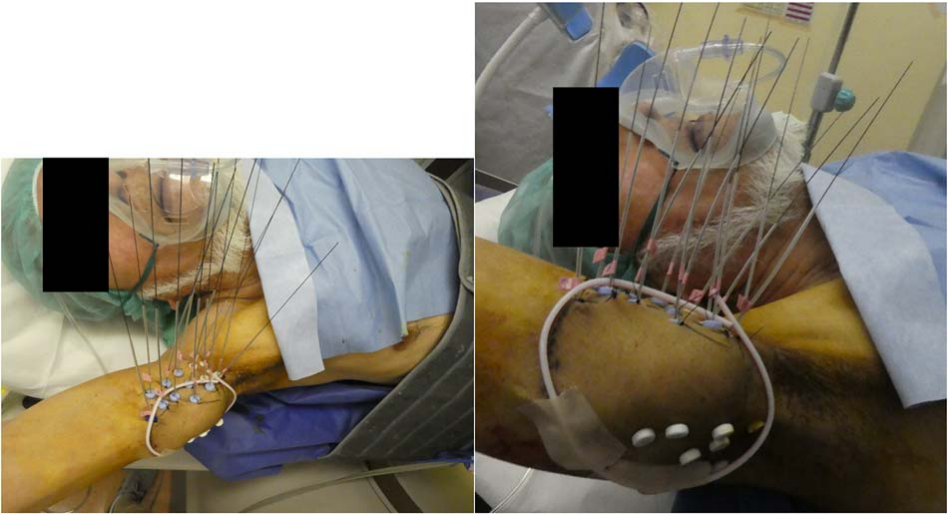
Under axillary brachial plexus blockade, evenly spaced 14 plastic interstitial needles were inserted parallelly guided by ultrasound so as not to injure the brachial artery. The needles were fixed by buttons attached to the needles and sutured to the skin. To aid the CTV contouring on the planning CT, which was set adding 1.5 cm around the GTV, a radiopaque marker was placed on the skin.
